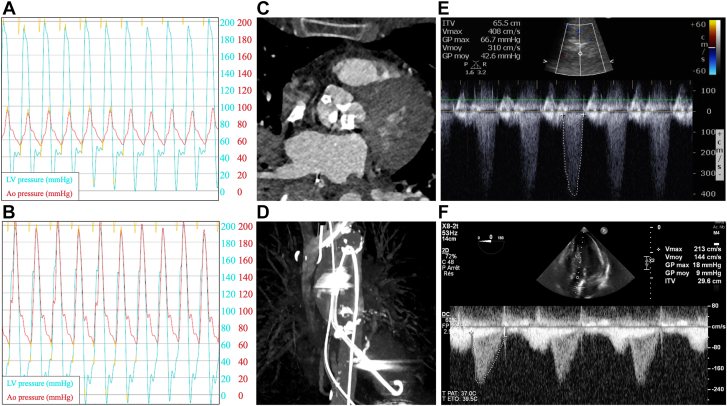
Hemodynamic and imaging data during Impella-supported bridge-to-TAVR. (A) Pre-Impella invasive pressure tracings showing severe peak-to-peak gradient of 125 mm Hg. (B) Post-Impella pressure tracings demonstrating immediate gradient reduction to 6 mm Hg. (C) Axial and (D) maximum intensity projection cardiac computed tomography with Impella CP correctly positioned across aortic valve into left ventricle. (E) Pre-TAVR transthoracic echocardiography showing a low-flow high-gradient aortic stenosis. (F) Post-TAVR transthoracic echocardiography showing complete recovery, with ejection fraction of 65%-70% and normal prosthetic valve function. TAVR, transcatheter aortic valve replacement.
